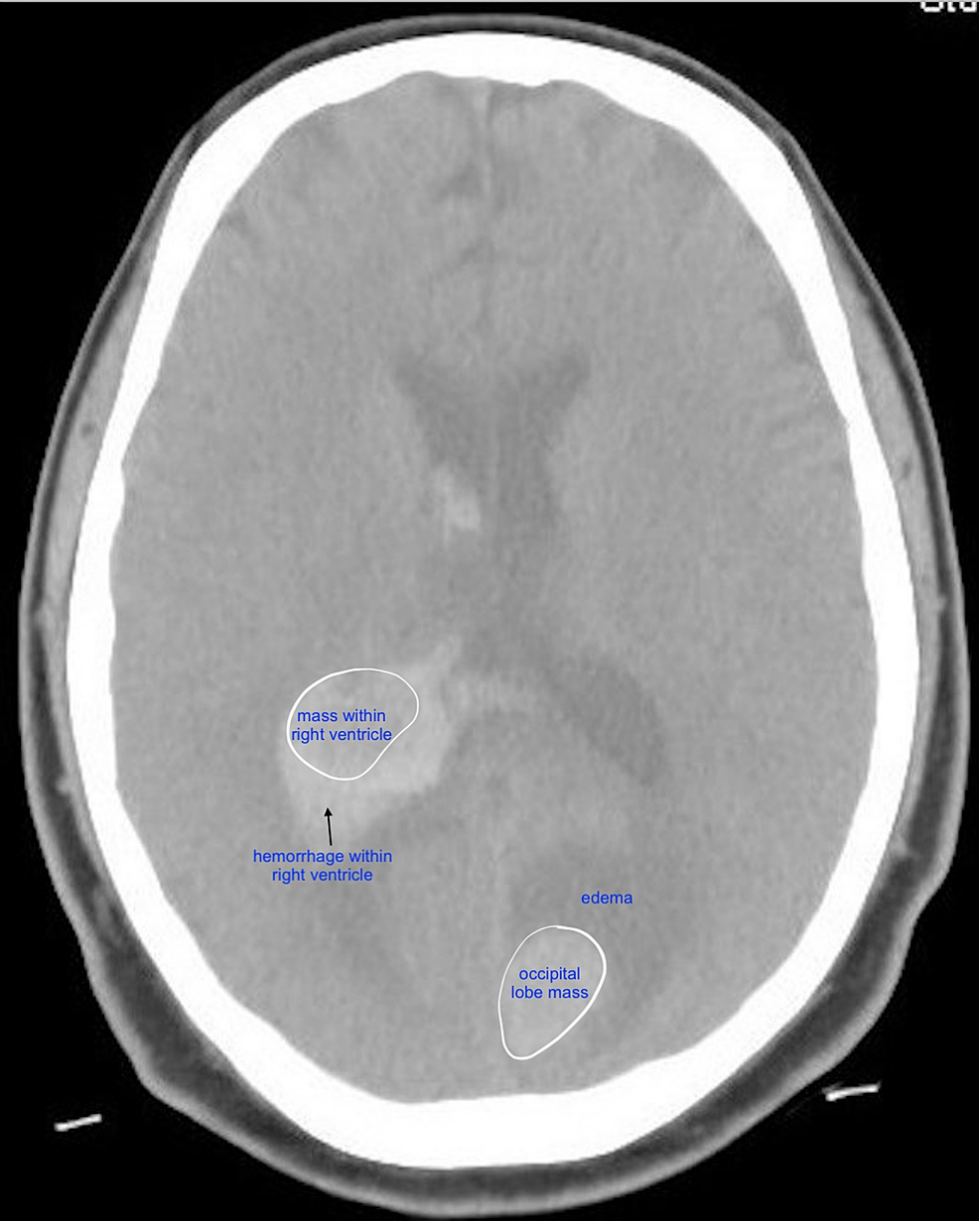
Non-contrast computed tomography (CT) scan demonstrating a 2 cm focal hyperdensity in the medial left occipital lobe, with appearance of intraparenchymal hemorrhage but suspicious for an underlying mass given the surrounding edema.
